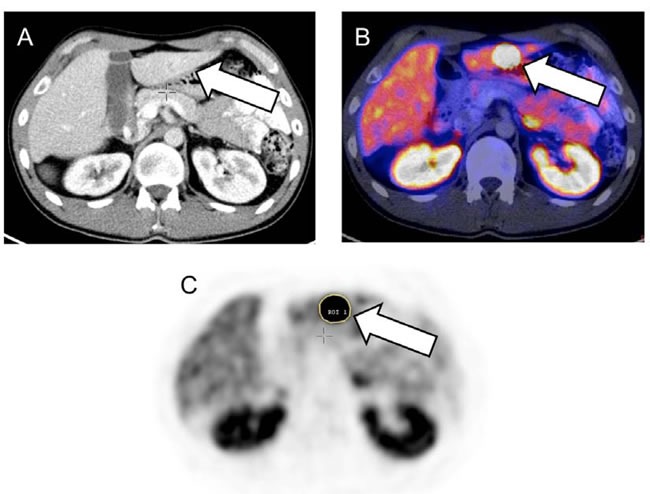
Baseline somatostatin receptor (SSTR) positron emission tomography/computed tomography (PET/CT) of a 24-year old male suffering from metastatic ileum neuroendocrine tumor Liver metastasis can be detected on CT (A) and on SSTR-PET/CT (B) indicated by the arrows. Manual stepwise segmentation of the lesion (arrow) by a region of interest on the PET-only images was performed (C). An overview of investigated heterogeneity parameters can be found in [26, 27], Table 4 and Figure 3.2.
